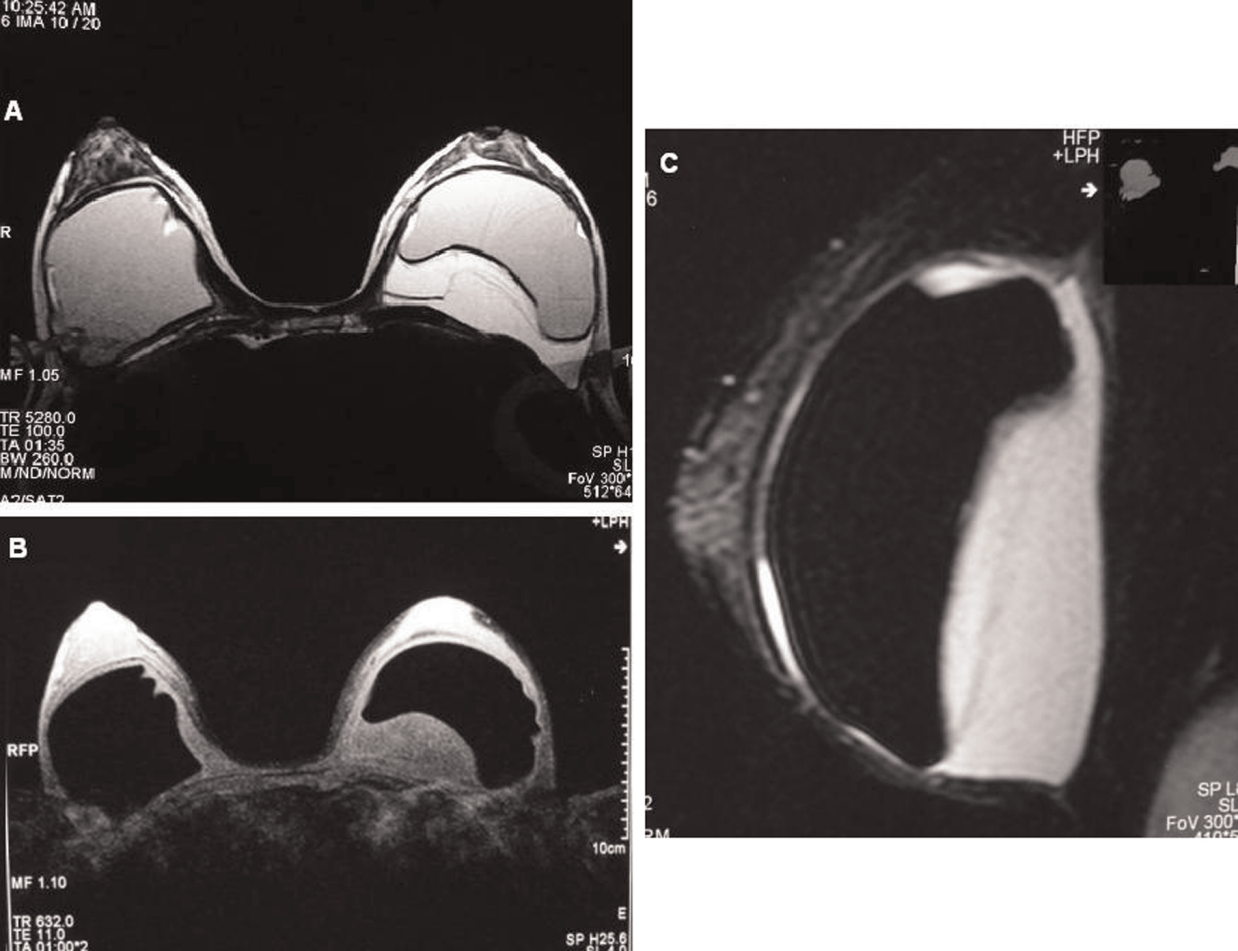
MRI examination of a retromammary seroma. (A) Axial T2-weighted image showing retroprosthetic collection with high signal intensity in the left breast. The silicone implant is intact. (B) Axial T1, with fat and silicone suppression, showing seroma with low signal intensity. Note the normal appearance of the right breast implant. (C) On sagittal STIR image with silicon suppression, the fluid collection shows high signal intensity.
